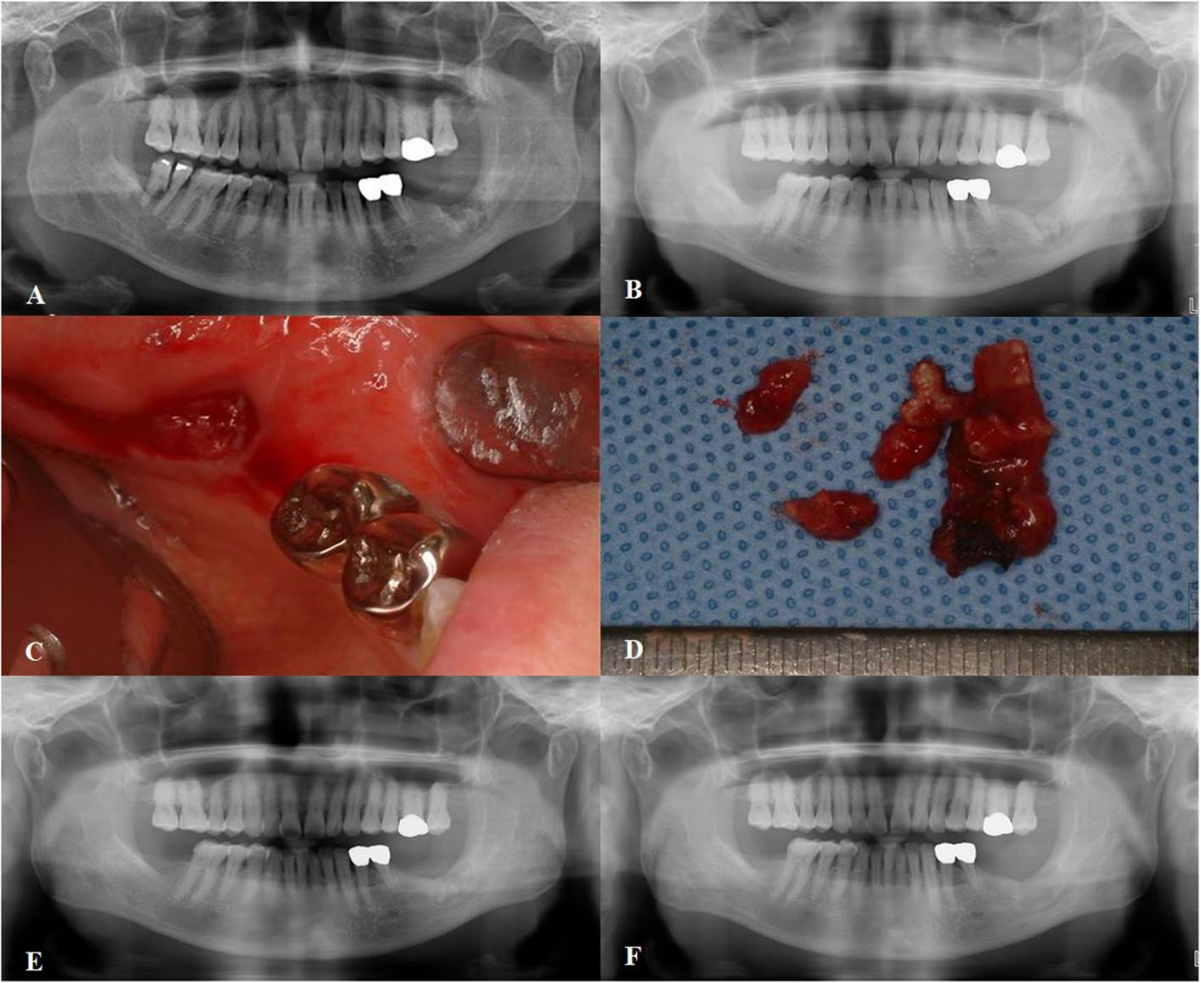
Surgical treatment of medication-related osteonecrosis of the jaw in osteoporosis patient. A Preoperative panoramic radiograph showing sequestrum formation in #37 extraction socket. B Panoramic radiograph of 3 months after antibiotic treatment. C Inflammation at #37 extraction site. D Removed necrotic bone after saucerization and sequestrectomy. E Panoramic radiograph of 3 years after antibiotic treatment showing the remaining sequestrum in #37 extraction socket. F Postoperative panoramic radiograph after 2 weeks
